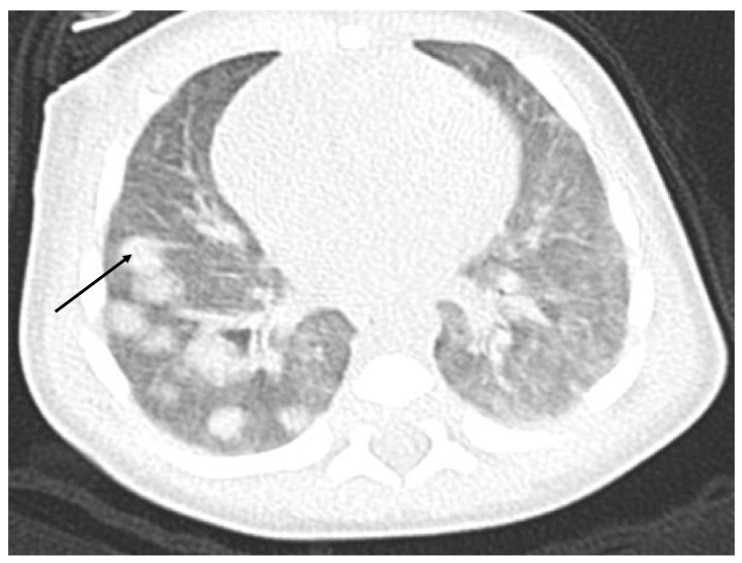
Chest CT with numerous metastatic lesions in both lungs.
